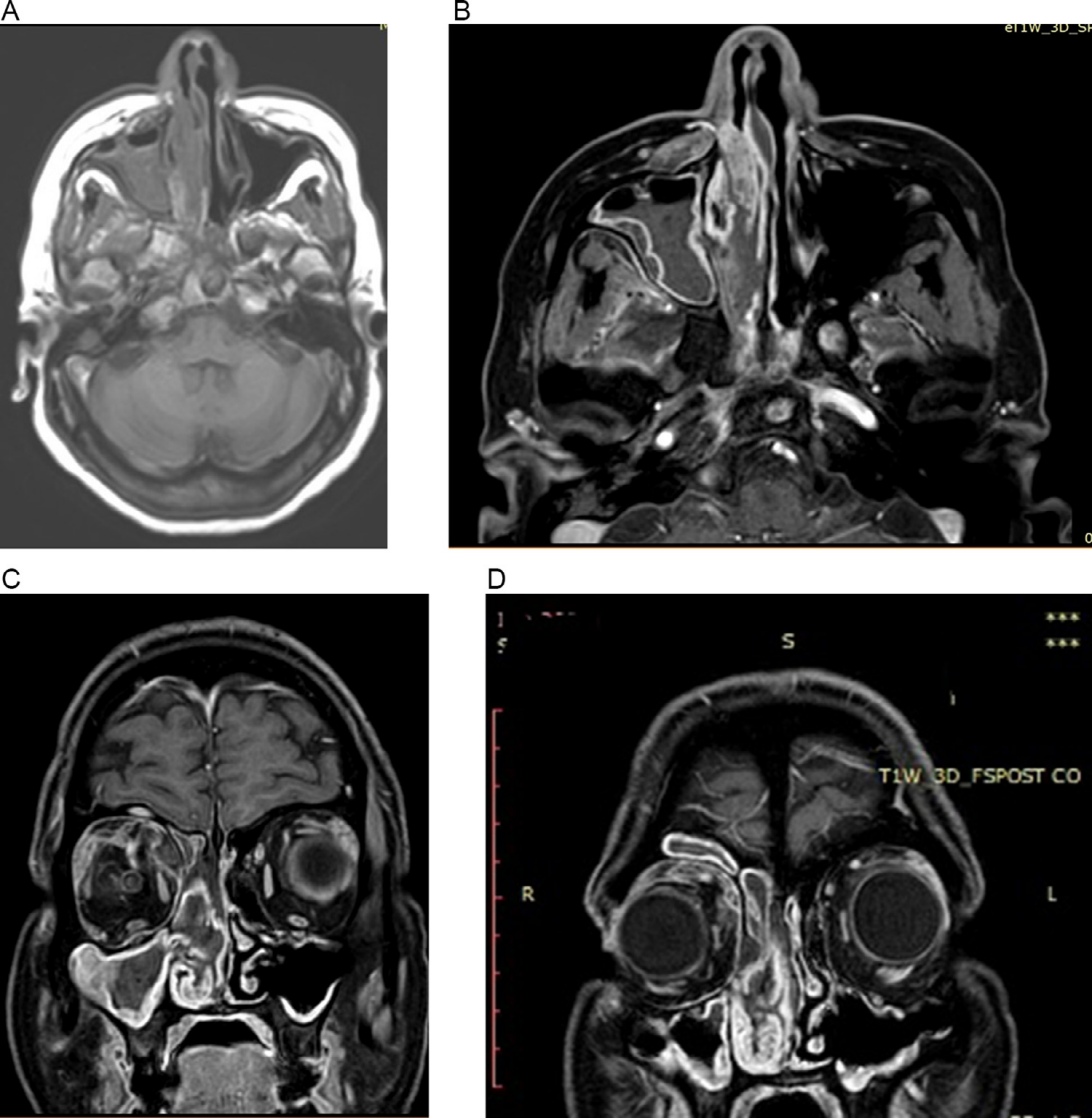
Magnetic resonance imaging (MRI) of coronavirus-associated mucormycosis. (A) T1WI axial section showing hypointense right maxillary sinus and right nasal cavity. (B) Post contrast MRI axial section showing enhanced inflamed mucosal lining of right maxillary sinus and nasal cavity. (C) Post contrast MRI coronal section showing retro-orbital involvement. (D) Post contrast MRI coronal section showing inflamed mucosal lining of right frontal sinus.
